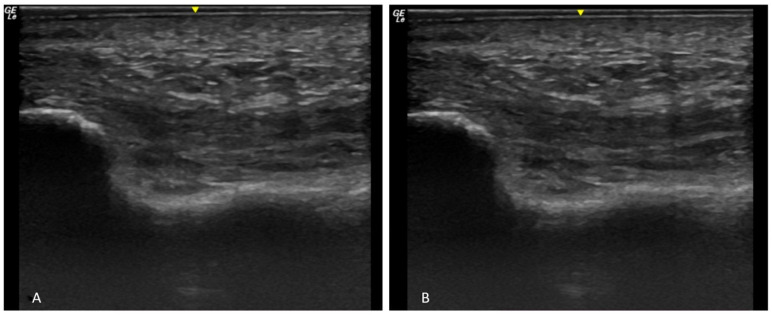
Representative longitudinal ultrasound images of a participant classified as recovered according to the predefined fibrillar pattern criteria. (A) Baseline ultrasound image showing disruption of the normal fibrillar architecture and diffuse hypoechogenicity in a plantar aponeurosis with flat morphology. (B) Ultrasound image obtained at the 52-week follow-up demonstrating recovery of the fibrillar pattern compared with baseline. According to the predefined ultrasound criteria, this case was classified as recovered.
